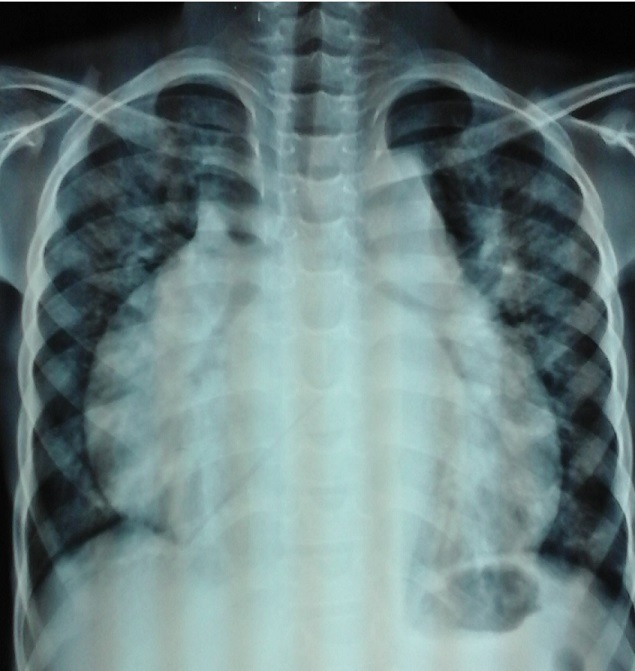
Radiographie du Thorax de Face: cardiomégalie et comblement de l?arc moyen gauche du c?ur
